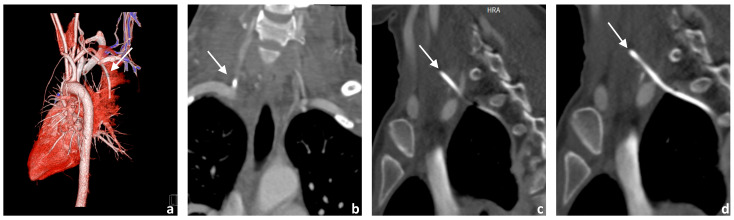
Imaging findings of the patient at initial presentation (a–d): 3D reconstruction of the CT angiography and supra-aortic vessels (a); CT angiography reformats of the course of the right vertebral artery in coronary (b) and sagittal orientation (c,d). The course of the incorrectly inserted Broviac catheter is indicated by arrows. The Broviac catheter inserted incorrectly into the V0 or V1 segment of the right vertebral artery ends with its tip in the pleural cavity.
